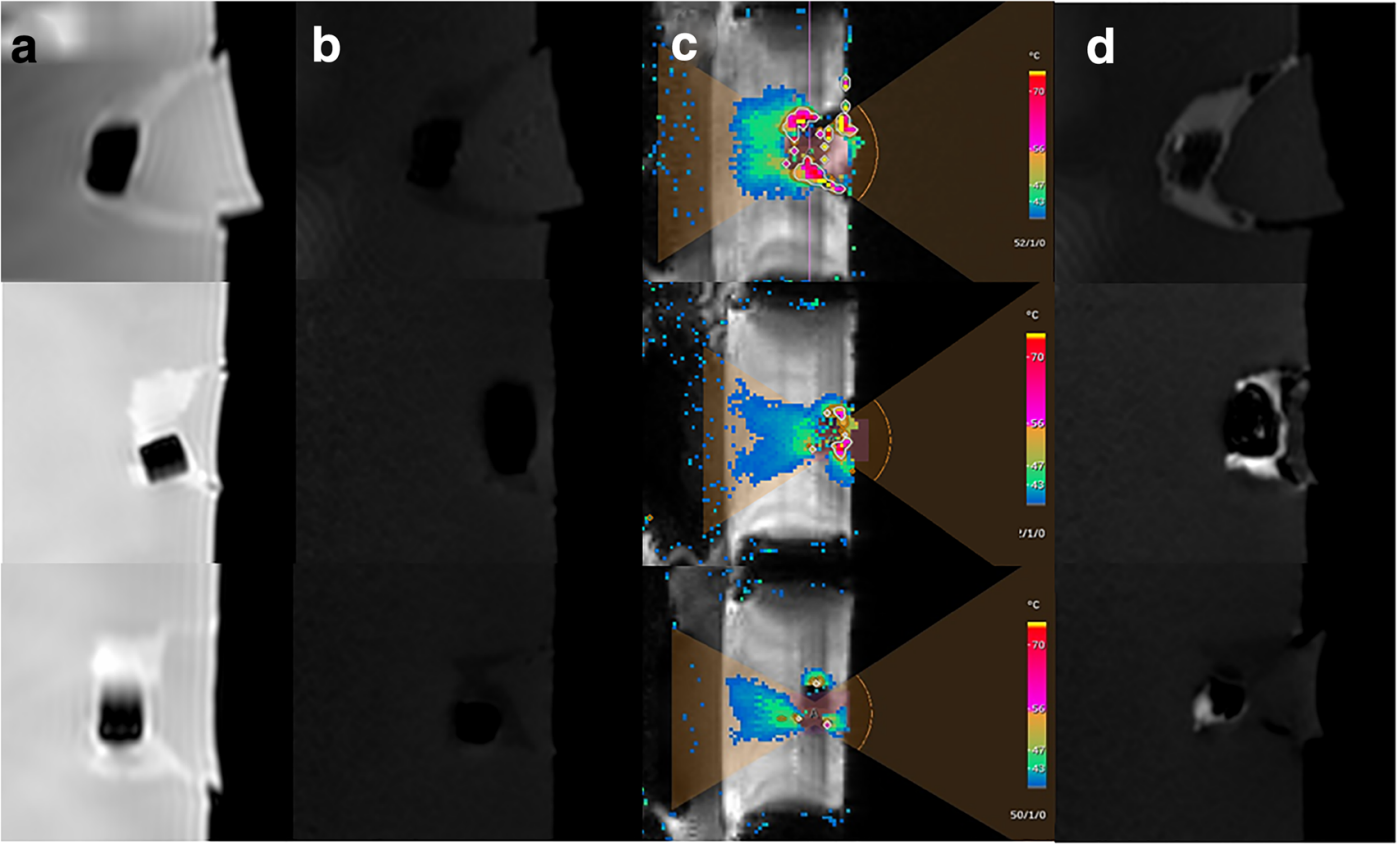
1.5-T MRI-tracked HIFU-triggered release of three wax-coated capsules (*n* = 3, separate experiments shown on three different layers). The position of the wax-coated capsule prior to the application of the HIFU pulse was determined using a 3D T2-weighted turbo spin echo sequence (repetition time 1000 ms, echo time 130 ms, a). The intact wax-coated capsule showed no hyperintensity on a 3D T1-weighted fast field echo sequence (repetition time 6.75 ms, echo time 3.3 ms, flip angle 10°), demonstrating that the non-hydrated lyophilised GBCA is not T1-hyperintense (b). T2-weighted MRI was used for guidance of the HIFU pulse and MR thermometry (colour mapping reveals thermal exposure, c). After the highly localised application of a 200-W HIFU pulse, a distinct T1-hyperintense signal was observed (d) using the same sequence as in b. The local temperature increase most probably melted the wax coating, allowing water to penetrate the capsule core and hydrate the encapsulated lyophilised GBCA, and thus resulting in the T1-hyperintensity. Hyperintensity varies depending also on the dissolution kinetics of the GBCA in the surrounding water, the amount of surrounding medium, and the imaging delay after HIFU-induced disintegration. GBCA: Gadolinium-based contrast agent; HIFU: High-intensity focused ultrasound
